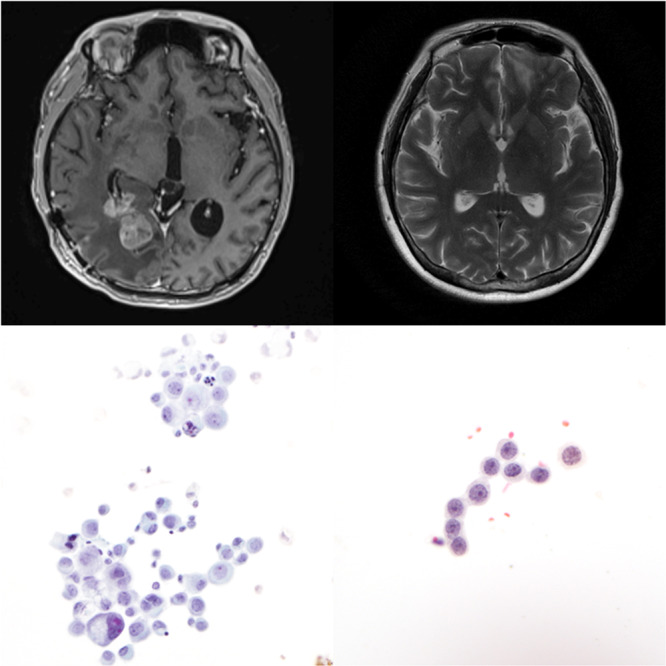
Radiological and cytological examples of leptomeningeal metastasis. Magnetic resonance imaging showing (a/top left) metastatic lung carcinoma at the right temporo‐occipital lobe with midline shift and abutting the cisterns and (b/top right) metastatic breast carcinoma presenting as an extra‐axial nodule at inferior left frontal lobe. Cerebrospinal fluid cytology showing (c/bottom left) pleomorphic vacuolated malignant cells in metastatic lung carcinoma and (d/bottom right) a hyperchromatic cluster of tumor cells in metastatic breast carcinoma.
